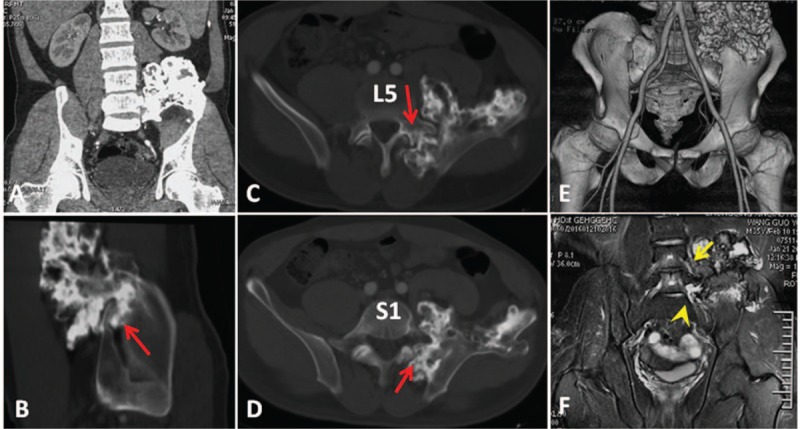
(A) Preoperative coronal, (B) sagittal, and (C, D) axial CT scans showing that the tumor (red arrow) involves the ilium, left sacroiliac joint, left transverse process, pedicle, and vertebral body of L5 and the sacrum. (E) The anterior aspect of the preoperative 3-dimensional computed tomography scan displays the proximity of the iliac vessels and the tumor. (F) Magnetic resonance image demonstrating that the tumor is adhesive to the L4 (yellow arrow) and L5 (yellow arrow head) nerve roots.
